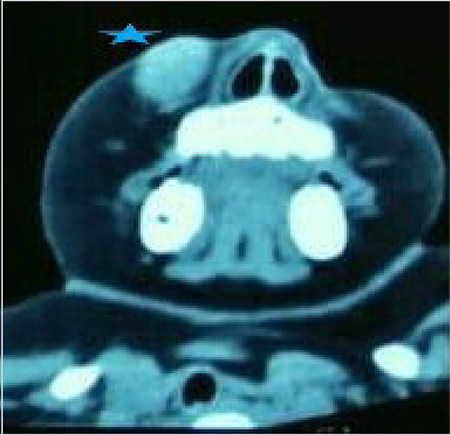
Contrast enhanced CT imaging showed an enhancing well-defined ovoid mass (5-point star) on the right side of the face with no bony erosion CT: Computed tomography
